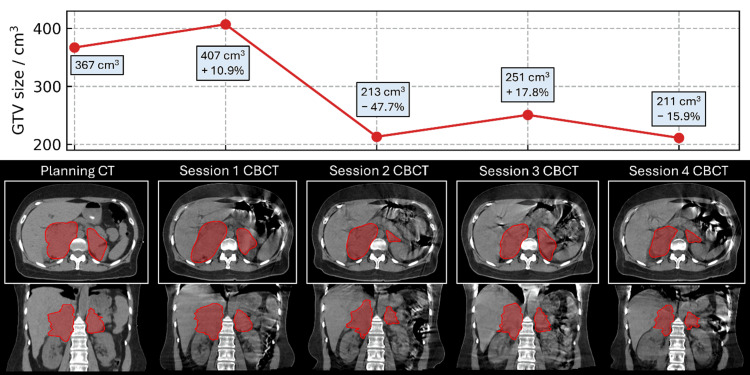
Progression of gross tumor volume (GTV) size throughout the course of treatment, where the percentage change for each timepoint is in reference to the previous session. The GTV contour is shown on the planning CT and the cone-beam CT (CBCT) for each of the four sessions. Treatments were delivered only for sessions 1 and 3.
